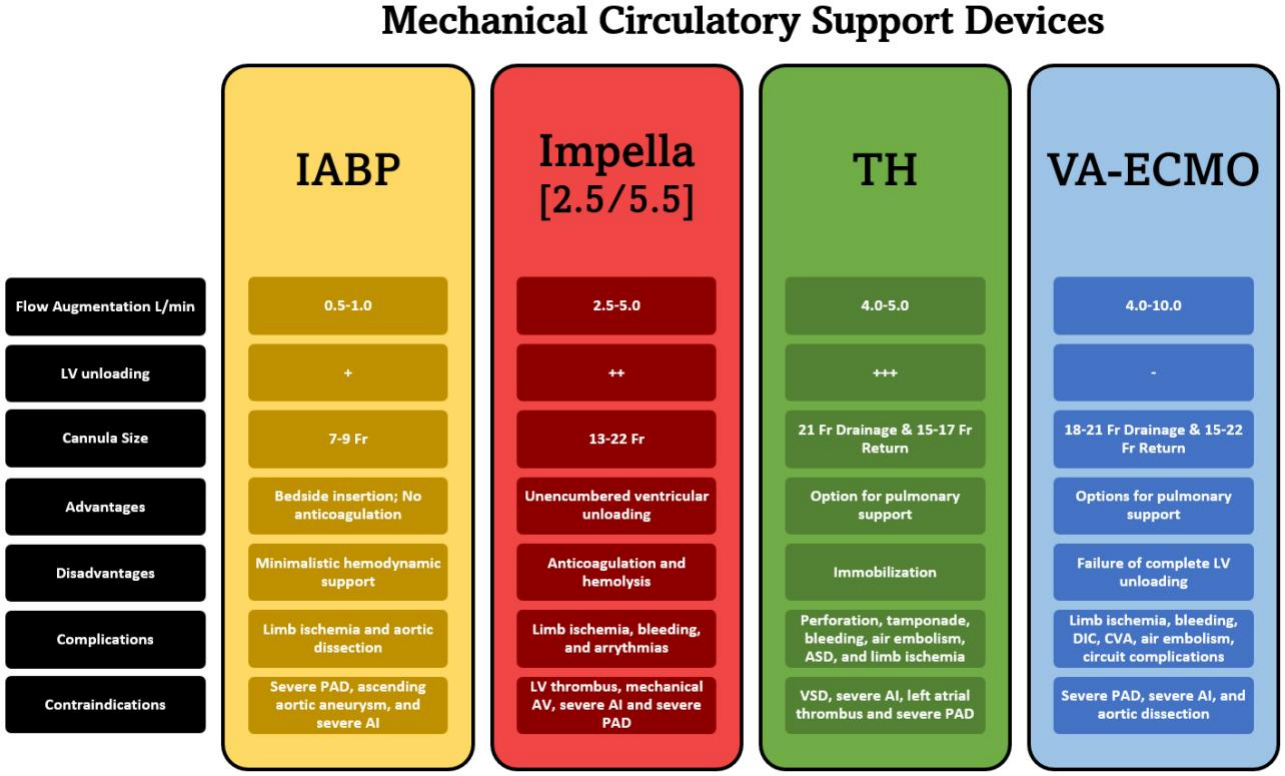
In severe AS with concomitant or impending CS, clear foresight of treatment methodology is necessary to circumvent a rapidly deteriorating state. Once TAVR is decided for definitive therapy, computed tomographic imaging is paramount to a successful procedural outcome via optimal valve selection and deployment. During this process, MCS can provide the left ventricular myocardium rest while allowing the heart team time to prepare the appropriate replacement valve. Among MCS options, the Impella® device may alter TAVR access site and result in significant artefact when performing valvular/vascular measurements which can affect valve sizing and planning. The Impella® lies in the aorta and is situated across the aortic valve. Depending on the patient’s physiology or physical limitations, this can pose the risk of entanglement or obstruction during the valve deployment process as the prosthesis is advanced with the Impella® in place up to the aortic valve. Further, the Impella® device must be removed while placing the TAVR, implying a period without support during valvular deployment. Contrary to this, the TH circuitry allows the patient to remain fully supported despite any haemodynamic perturbations that may occur while performing TAVR. Despite TAVR placement, most patients continue to require further MCS post deployment to allow for complete recovery—in the setting of Impella® that means placing an entirely new Impella CP catheter across the new prosthesis, a process that can predispose the prosthesis leaflet to unintentional damage. In fact, this necessitated a Class 1 FDA recall, followed by a review and update of the instructions for Impella® use. Alternatively, the TH can remain in position without adjustments of circuitry pre-, intra-, and post-TAVR, with adjustments of pump flow dictated by the patient’s clinical status. Finally, in general, the TH provides greater overall cardiovascular support than its counterpart, the Impella CP (*Table 1*), which ultimately enables both tailoring of flow and early de-escalation of vasopressors/inotropes. For these reasons, TH becomes the clear choice when deciding on MCS while contemplating TAVR (*Figure 2*). However, cons associated with this approach include large bore access, need for systemic anticoagulation, performance of acute trans-septal puncture, aggressive volume loading, high rate of transfusions, haemolysis, and acute limb ischaemia; these risks need to be tempered with the overall benefit before deciding on this approach and may be reduced by early valvular replacement to avoid the deleterious effects of the TH system which occur with prolonged use.
